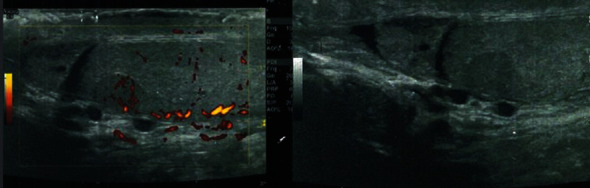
Scrotal ultrasound showing hypervascular hypoechogene testicular module measuring 8∗6∗8 mm.
